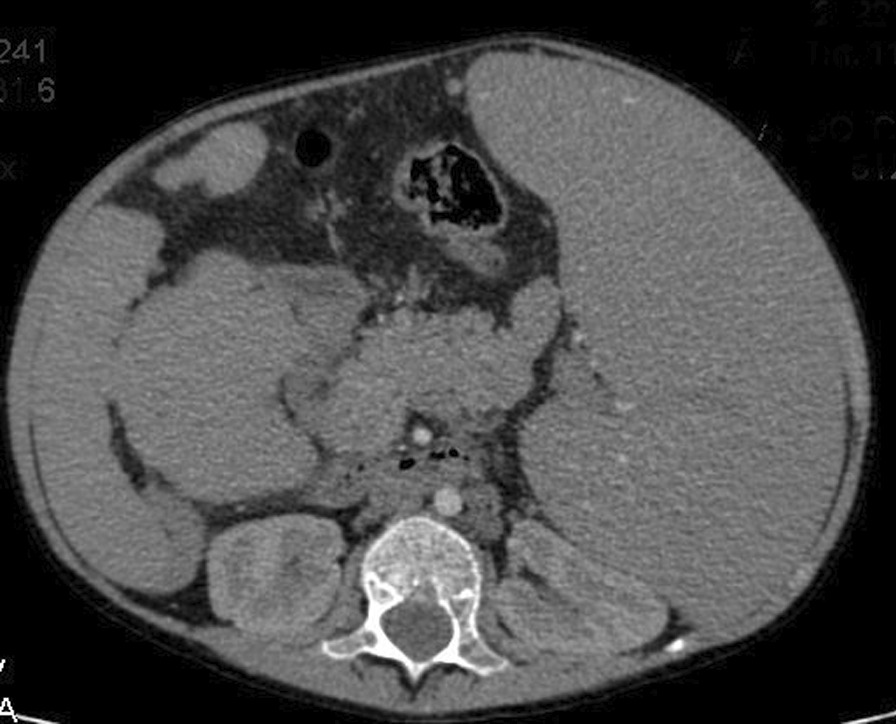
Abdominal CT scan with contrast showing splenomegaly
